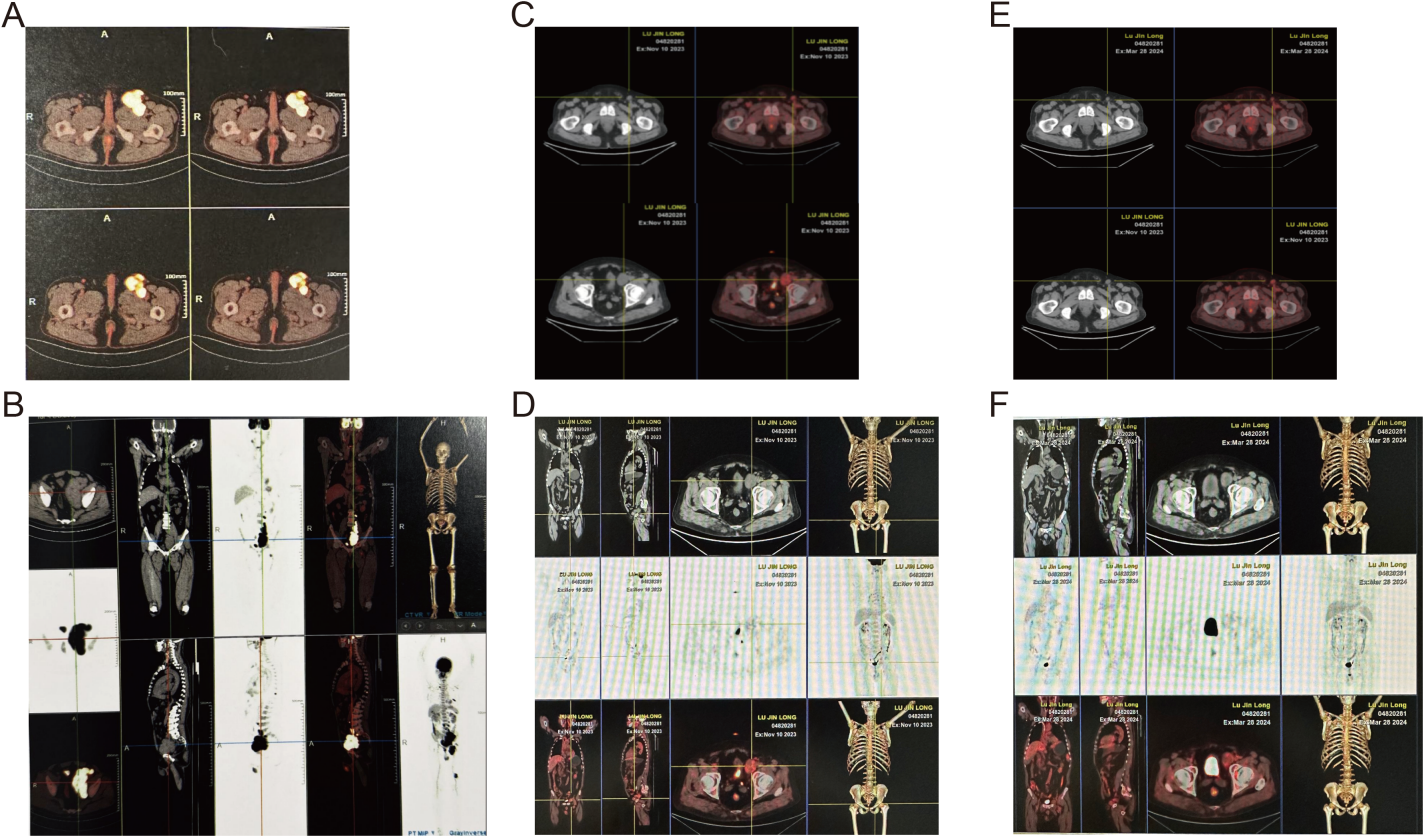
Whole-body ^18^F-FDG PET-CT assessment of disease extent and sequential therapeutic response. (A, B) Pre-treatment MIP (A) and matching axial pelvis image (B) showing widespread hyper-metabolic lymphadenopathy (SUVmax 15.9) and osteolytic destruction of the left ilium (SUVmax 14.6). (C, D) After four cycles of MVPD regimen the MIP (C) and axial pelvis image (D) reveal marked reduction in nodal size and metabolic activity (residual SUVmax 5.2; iliac lesion SUVmax 4.4). (E, F) One-month post-autologous stem-cell transplantation plus BCMA CAR-T infusion the MIP (E) and axial pelvis image (F) demonstrate complete metabolic resolution with no detectable abnormal uptake, consistent with hematologic complete remission and extra-medullary response > partial remission.
